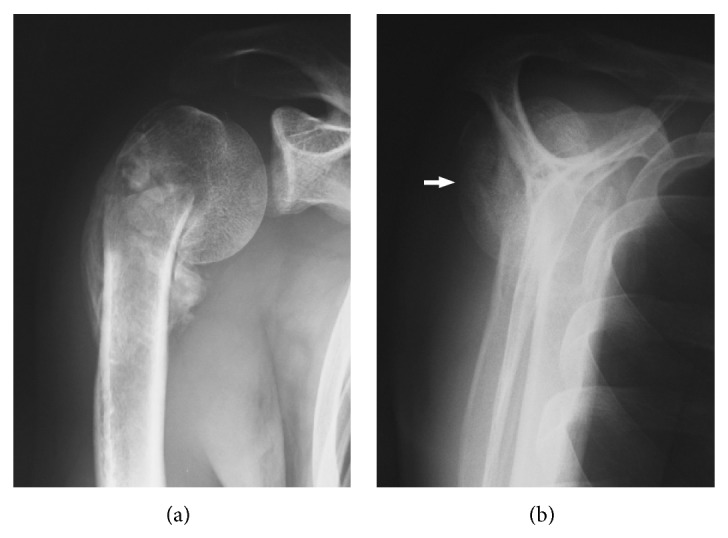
Preoperative anteroposterior (a) and scapula-Y (b) radiographs demonstrate varus and retroversion (arrow) deformity of the humeral neck.
